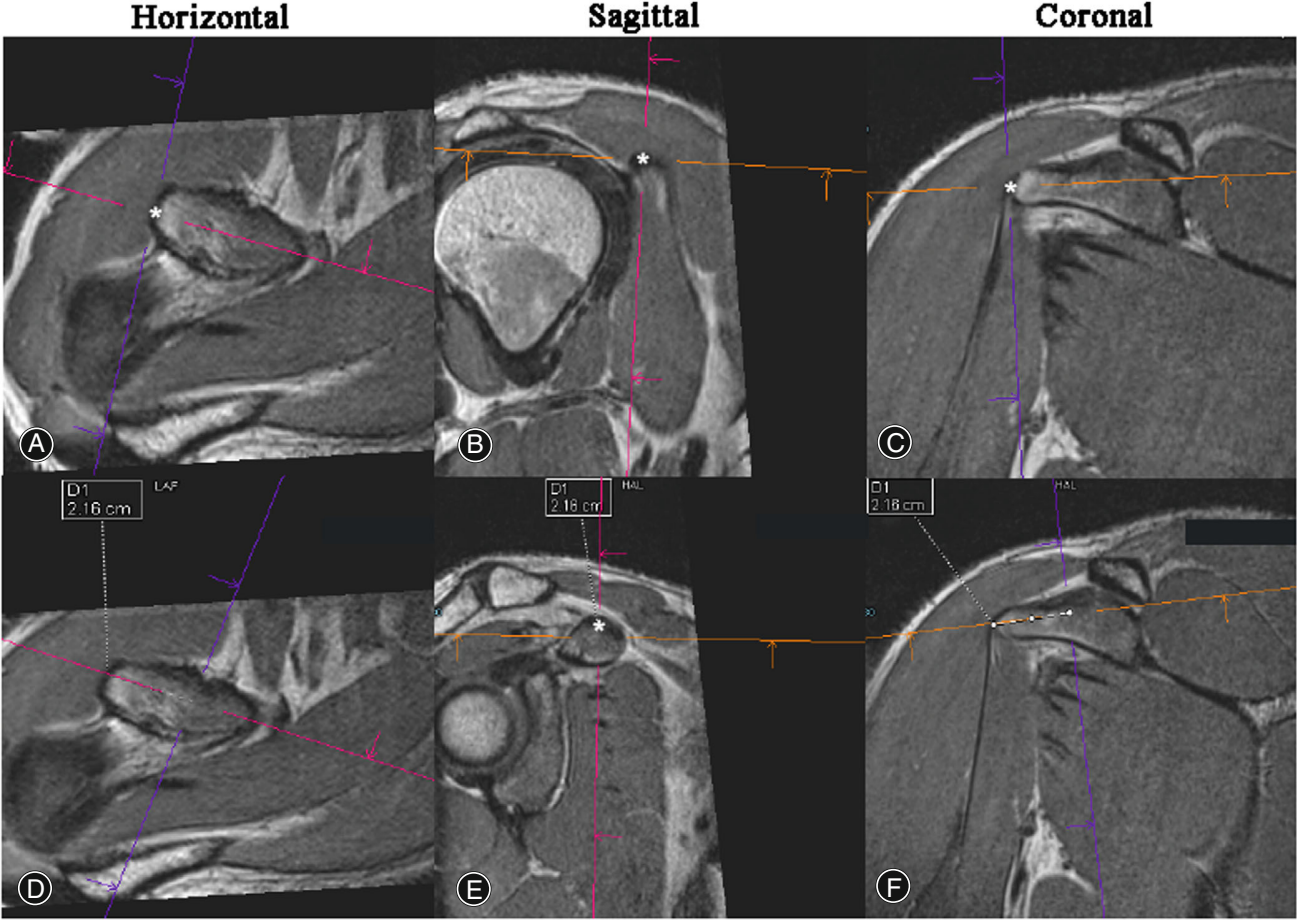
Data acquisition from 3D MRI PD‐SPACE‐COR imaging. The coracoid tip is identified as the point most distant from the base on the coracoid on the horizontal plane. The tip of the coracoid process has been localized with an asterisk in the horizontal (A), Sagittal (B), Coronal (C) planes. Meanwhile, the lateral edge of the attachment of trapezoid ligament (TL) has been localized with an asterisk in the horizontal plane (D) and sagittal plane (E). and, the predicted SM, namely, the distance between two asterisks (represent the coracoid tip and lateral edge of TL attachment) was measured (F).
